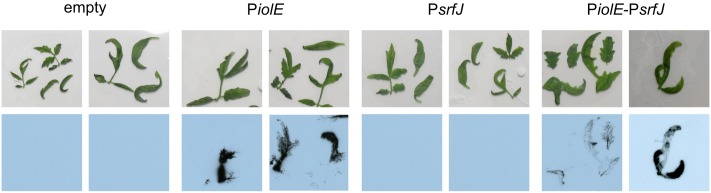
Expression in plants. *S.* Typhimurium strain 14028 carrying derivatives of plasmid pSB377 (empty, P*iolE*, P*srfJ*, P*iolE*–P*srfJ*) was used to spray 3-week-old tomato plants grown in sterile conditions. Plants were germinated in ½ MS for 1 week and grown in ¼ MS media during 2 weeks. Bacteria were grown 1 day before the infection in LM plates. Three tomato plants were spray-inoculated with bacteria suspended in 10 mM MgCl_2_ at OD_600_ 0.1. Tomato plants were imaged 2 days p.i. with an X-ray film exposed for 48 h.
